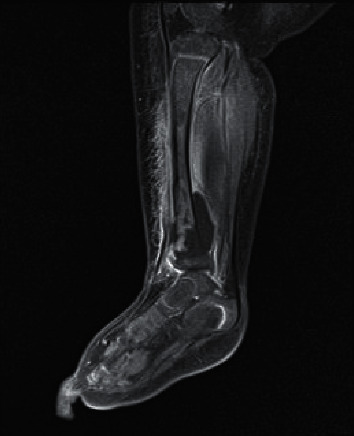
Osteomyelitis changes in the right tibial region by MRI.
